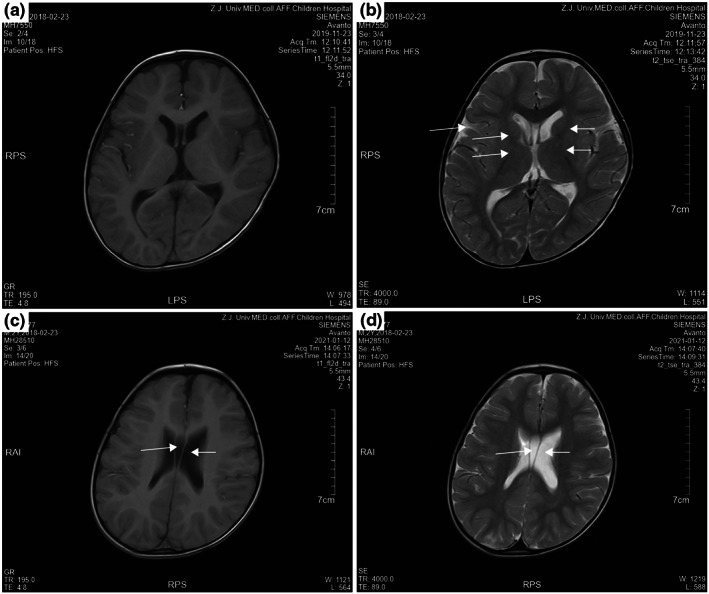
MRI results in our patient in 2019 (a, b) and 2021 (c, d). (a) T1‐weighted axial image. (b) T2‐weighted axial images. The widened extracerebral space and the internal capsule showed less signal reduction. (c, d) T1‐ and T2‐weighted axial images showed a widened extracerebral space and lucid septum cysts.
